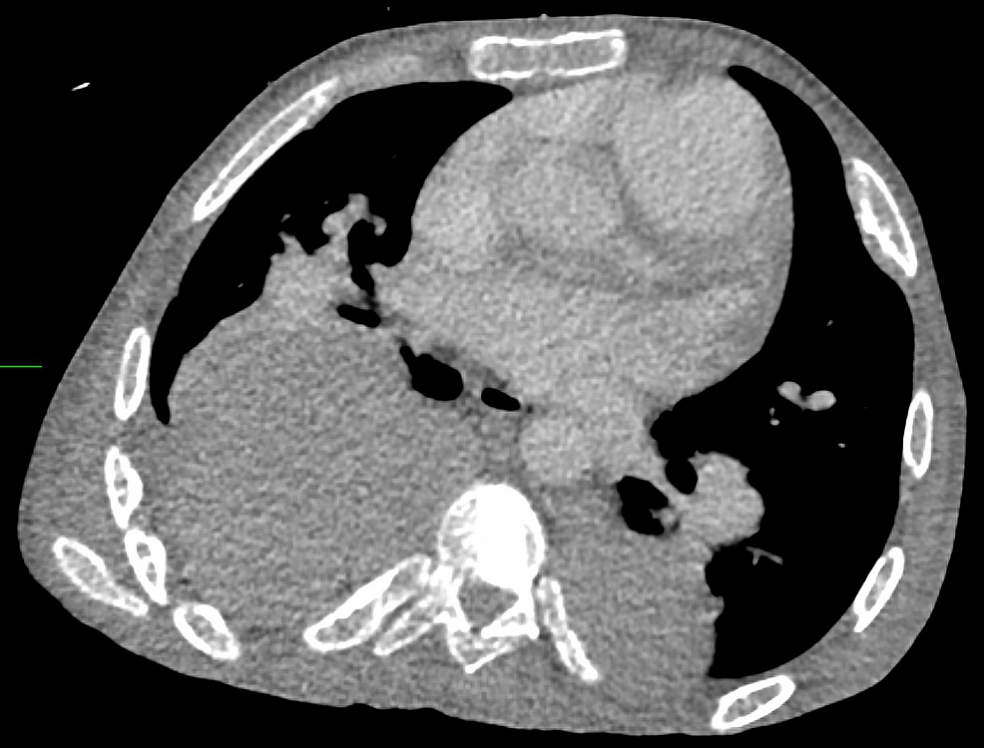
Computed Tomography of the Thorax: Axial plane view demonstrating posterior paraspinal masses, particularly a large right posterior paraspinal mass.
